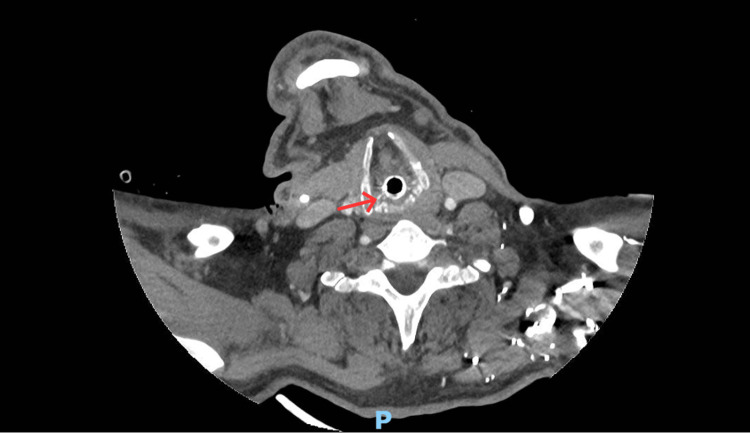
Axial CT of the neck demonstrating vocal cord and paraglottic oedema with the endotracheal tube in situ. The red arrow highlights oedematous thickening of the vocal cord and paraglottic soft tissues, associated with narrowing of the laryngeal airway.
